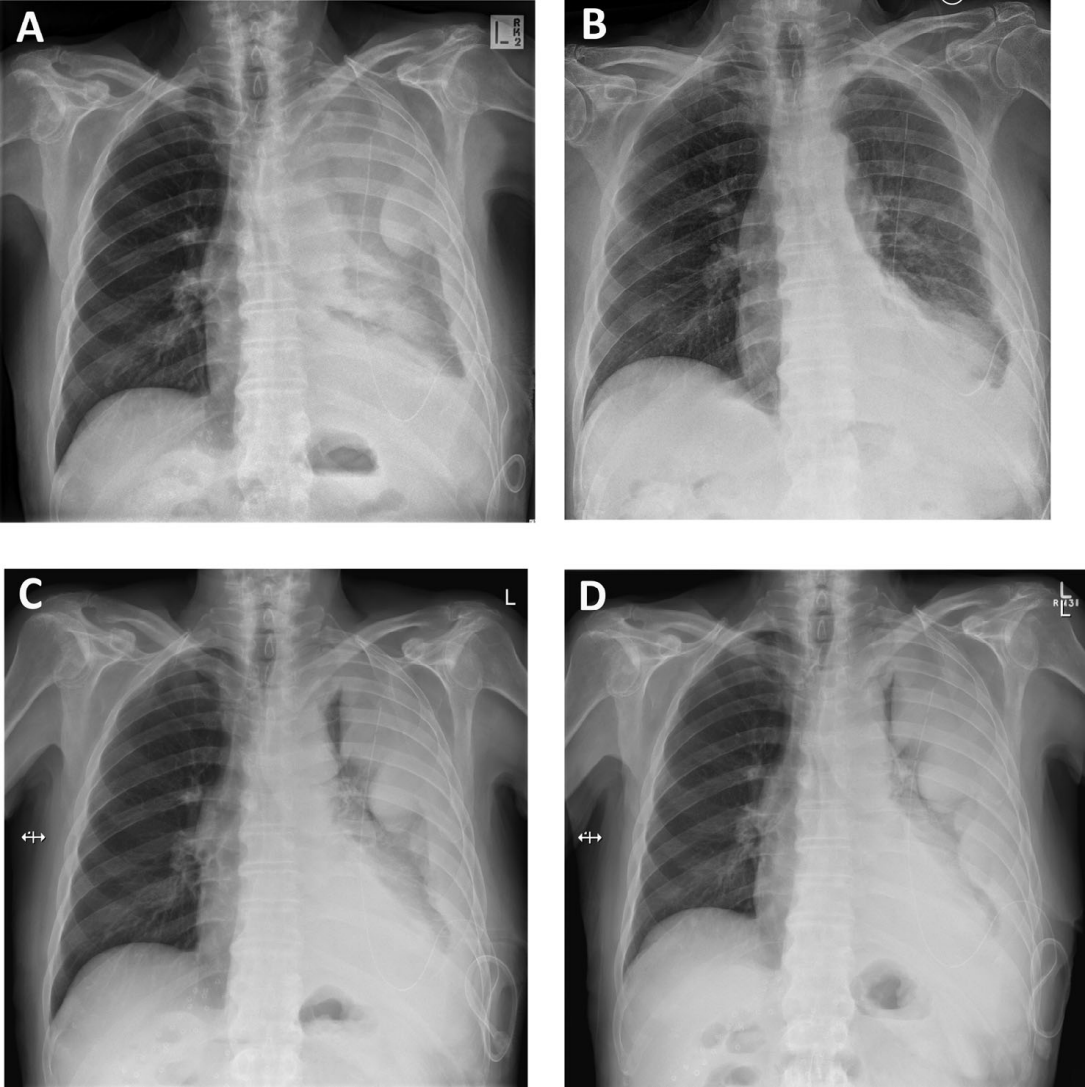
The viscous fluid of Patient 1 made drainage via indwelling pleural catheter (IPC) difficult, resulting in large loculated collections of pleural fluid, requiring hospital admission. The chest radiograph (A) showed almost complete opacification of the left hemi‐thorax which cleared with intrapleural tissue plasminogen activator (tPA) and deoxyribonuclease (DNase) therapy (B). However, the benefit was short‐lived and significant re‐accumulation of fluid was shown on his radiograph two weeks later (C). Further treatment with escalated doses of tPA/DNase failed to produce a response (D).
